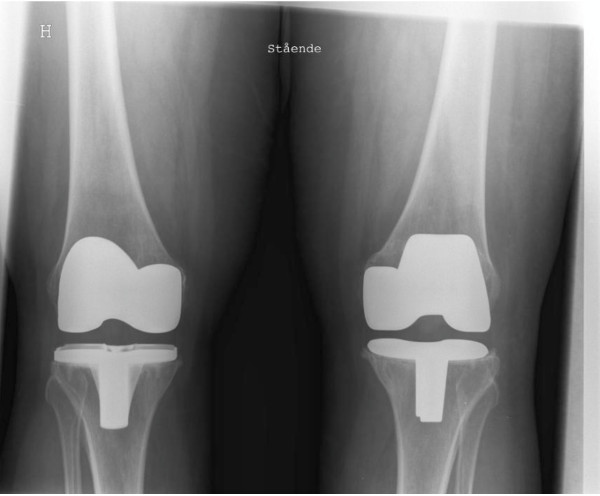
Anteroposterior radiograph showing the knees of a standing patient 1 year postoperatively. H: Right knee, LPS-Flex (Zimmer^®^). Left knee, AGC (Biomet^®^).
